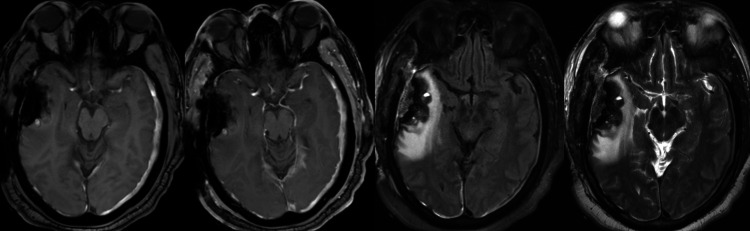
Pre- and post-contrast MRI status post-tumor resection (left to right: T1 pre- and post-contrast axial sequences, FLAIR sequence, and T2-weighted sequence). MRI demonstrating no evidence of residual enhancing tumor at the operative site with involution of the thickened, tumoral capsule and overall decreased mass effect.
